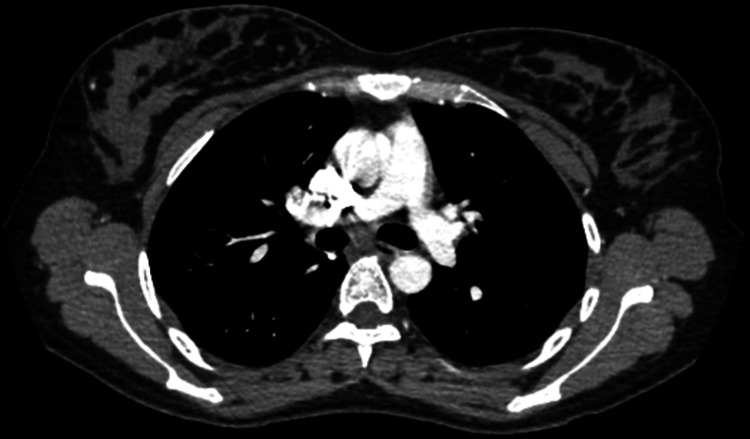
Axial CTA of PE workup with no apparent filling defects at the level of the pulmonary trunk bifurcation. There is some mild streak artefact from hyperdense contrast in the SVC. CTA - computed tomography angiography; SVC - superior vena cava
